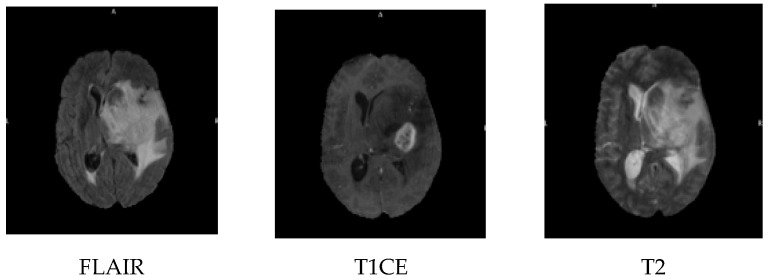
Magnetic resonance imaging sequences of the brain (left to right): FLAIR, T1CE, and T2 sequences.
